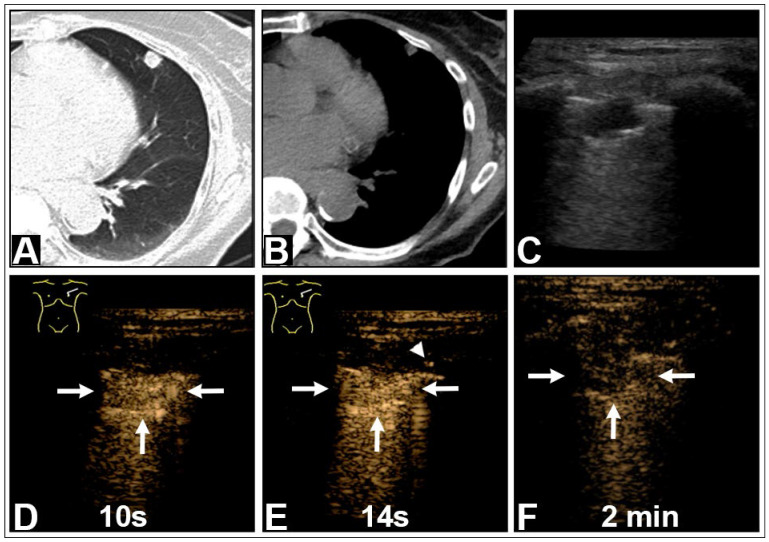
Representative biopsy-confirmed case of a 75-year-old female patient with a diagnosis of spindle cell sarcoma and pulmonary nodules on CT (A,B). On B-mode ultrasound (C), a hypoechoic pleura-adjacent pulmonary nodule was identified. On CEUS (D), the lesion demonstrated early enhancement after 10 s (arrows), preceding enhancement of the thoracic wall vessels, which became visible only after 14 s ((E), arrowhead). The lesion exhibited a marked and homogeneous enhancement pattern, consistent with a predominantly pulmonary-arterial supply. At approximately 2 min (F), an early decline in enhancement was observed, indicating rapid washout.
